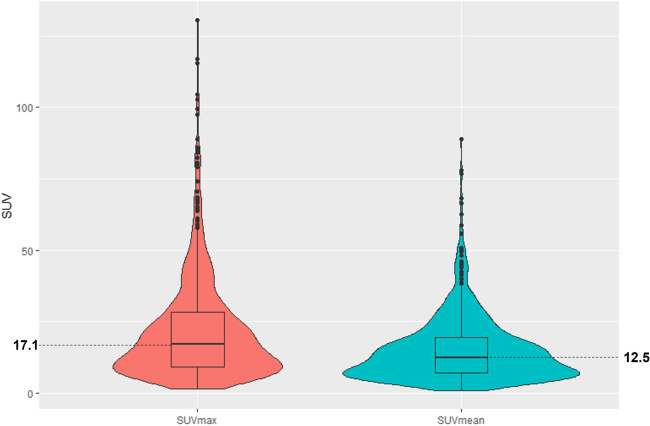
Quantitative assessment (*SUV*_*max*_ and *SUV*_*mean*_) of bladder activity
